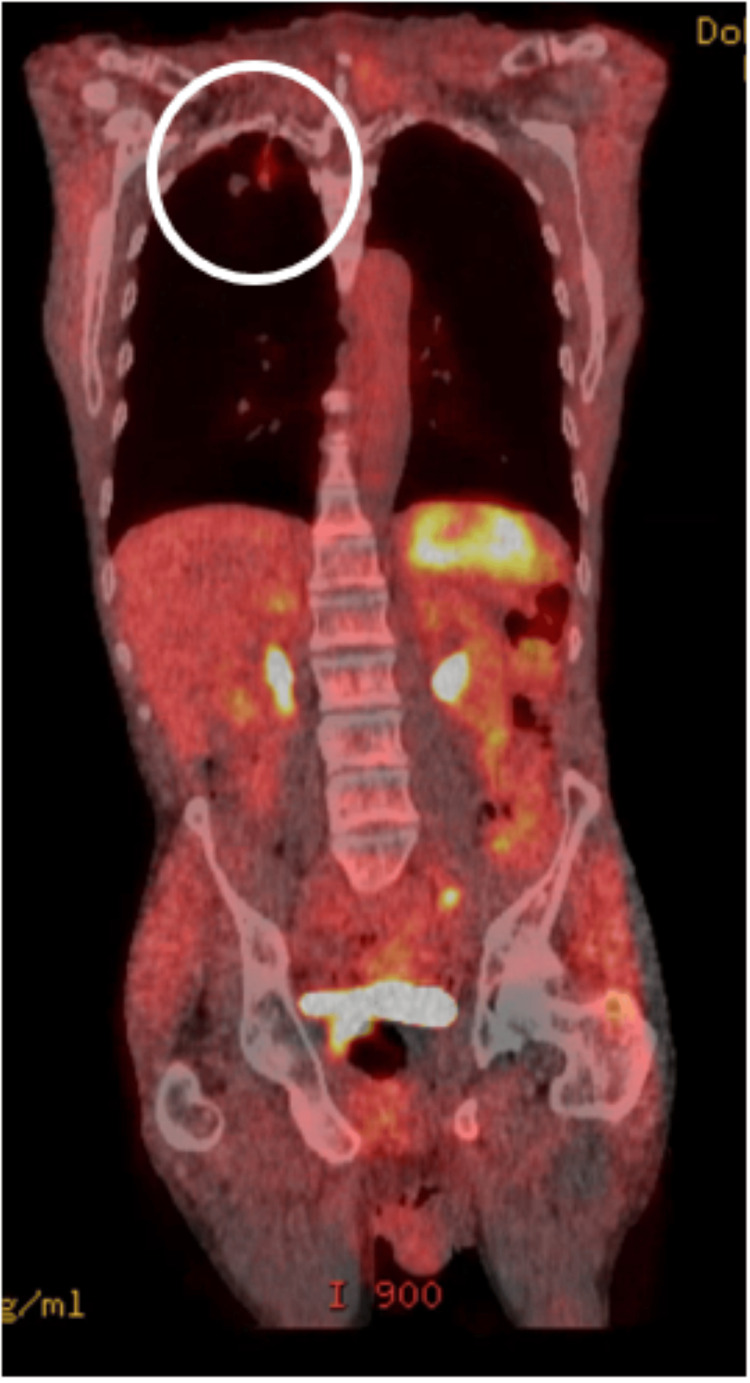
PET scan coronal view: right upper lobe nodule with SUVmax 4 PET: positron emission tomography; SUVmax: maximum standardized uptake value
